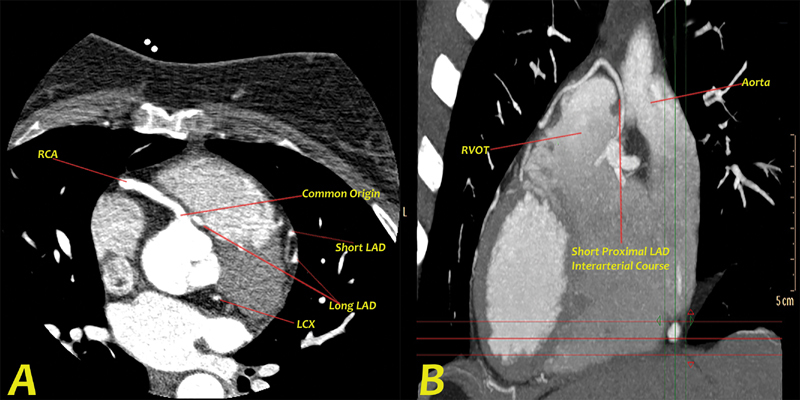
Computed tomography coronary angiography showing (
